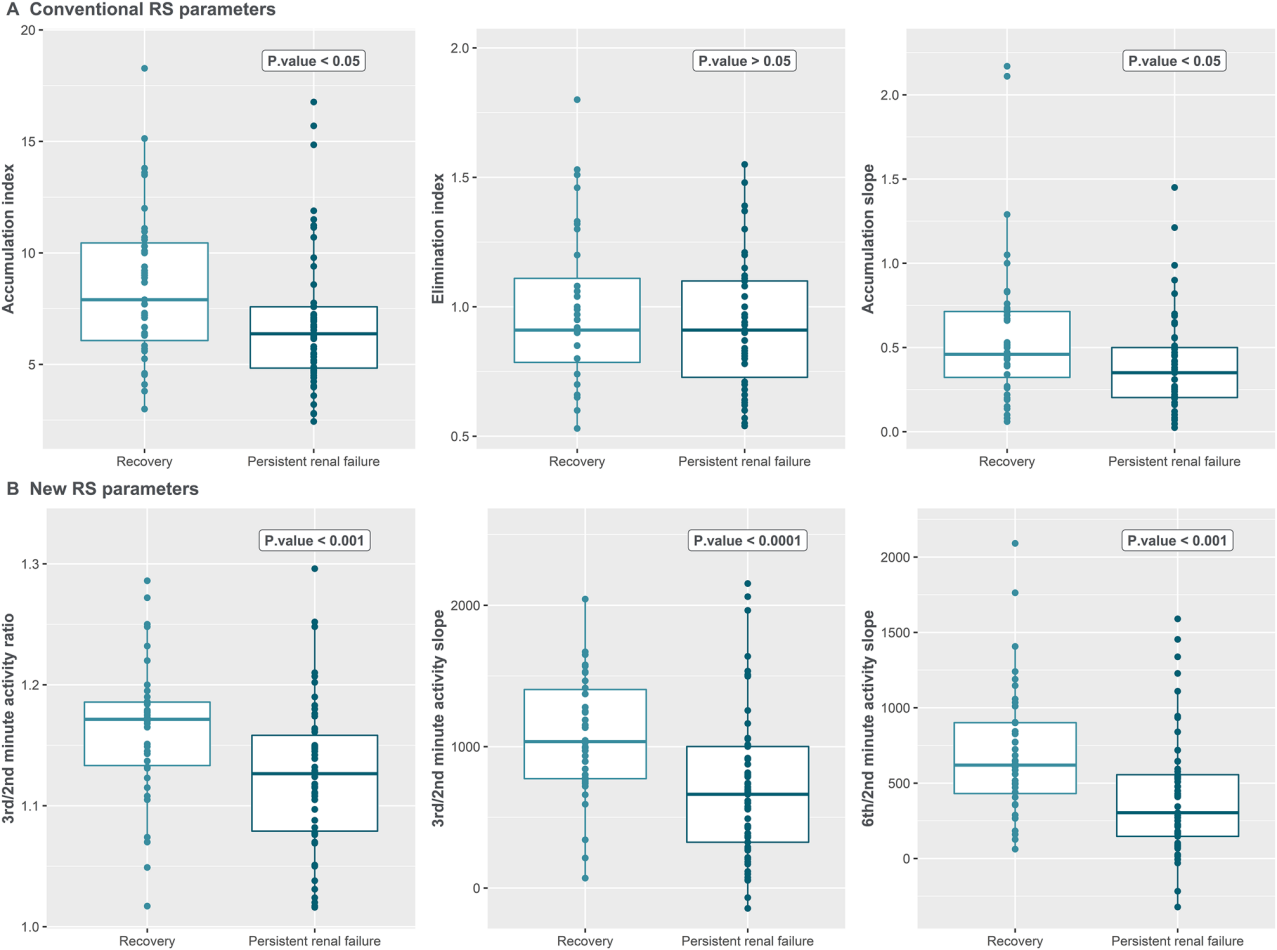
Renal scintigraphy parameters comparison by box-and-whiskers plot between renal recovery and persistent renal failure. *Min.* minute, *RS* renal scintigraphy. The lower and upper hinges correspond to the first and third quartiles. The middle hinge corresponds to the median. The upper whisker and lower whisker, respectively, extends from the upper and lower hinge to the largest and lowest value no further than 1.5 times the interquartile range. The sum of the accumulation index and accumulation slope of the two kidneys was considered in this analysis. For the other parameters, the values of the best kidney were considered. Outcome was missing for 6 patients (No creatinine available at baseline or in the follow-up period). Missing values for total accumulation index (1 patient), elimination index (2 patients), accumulation slope (3 patients)
